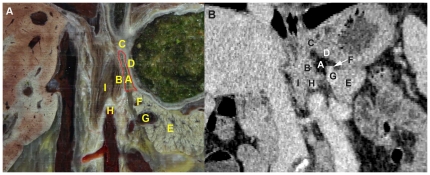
Presentation of the triangular-shaped LES on coronal MPR images from the front. (A, B) Visualization of the LES on CVH2 and CT images, respectively. A: LES; B: left crura of the diaphragm; C: gastric cardia; D: gastric fundus; E: pancreas; F: splenic artery; G: splenic vein; H: root of the celiac trunk; I: right crura of the diaphragm.
